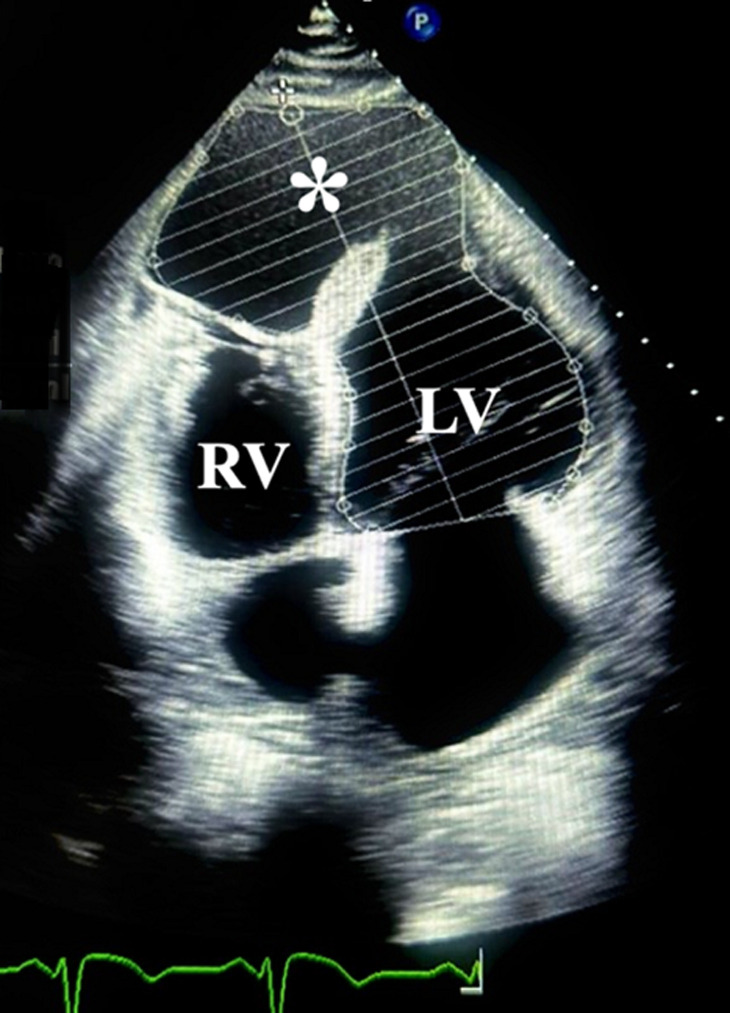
echocardiogram, 4-chamber view showing pseudoaneurysm (*) communicating with the left ventricle (LV), right ventricle (RV)
